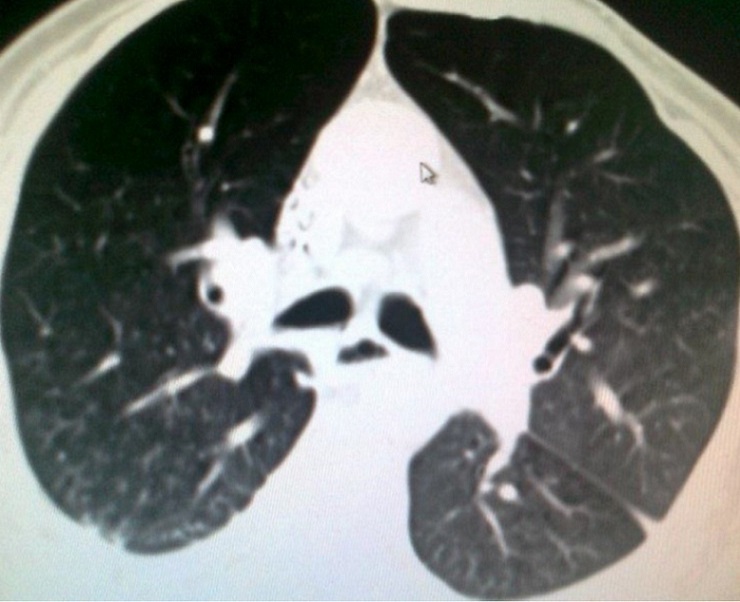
CT-scan chest with contrast
